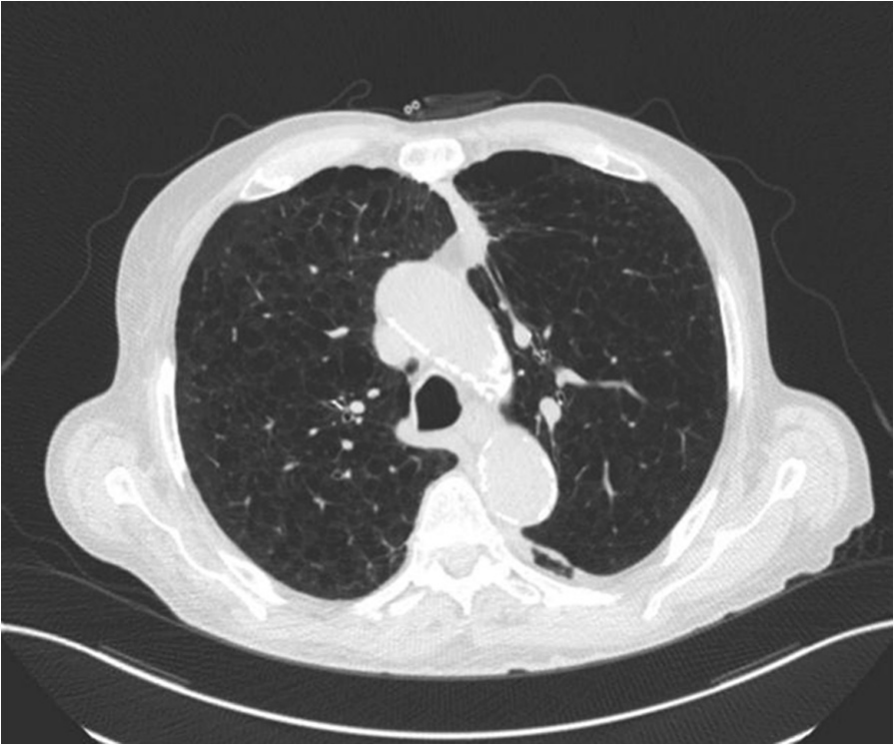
CT scan at 46-months of follow-up with residual consolidative mass at the site of the biopsied lesion.
